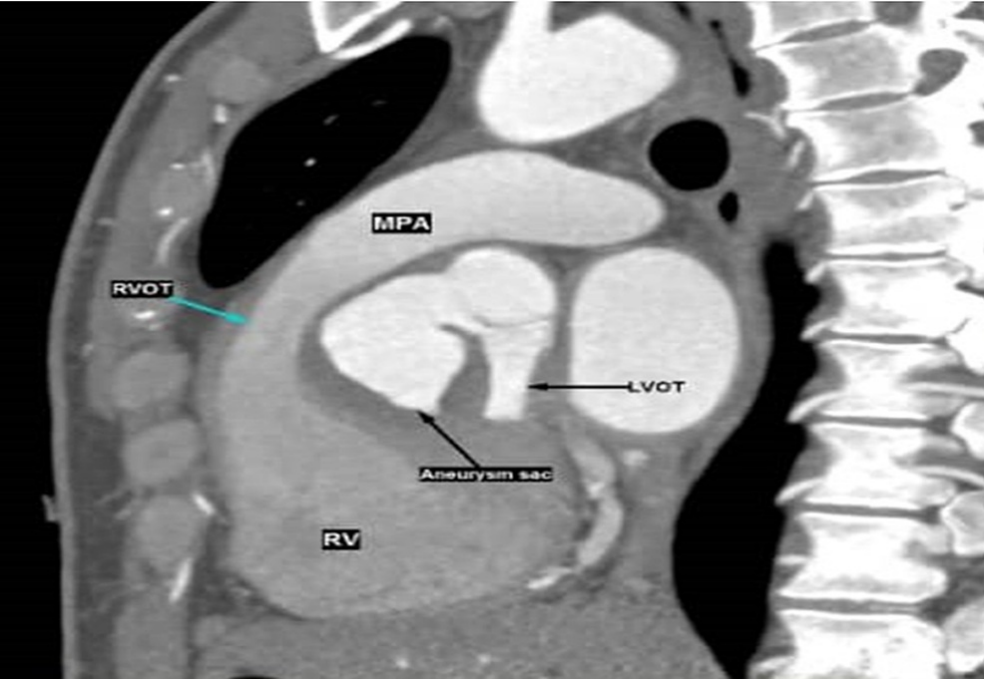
Cardiac CT scan shows the aneurysm sac with the compression effect to the right ventricular outflow tract. LVOT: left ventricular outflow tract; RV: right ventricle
